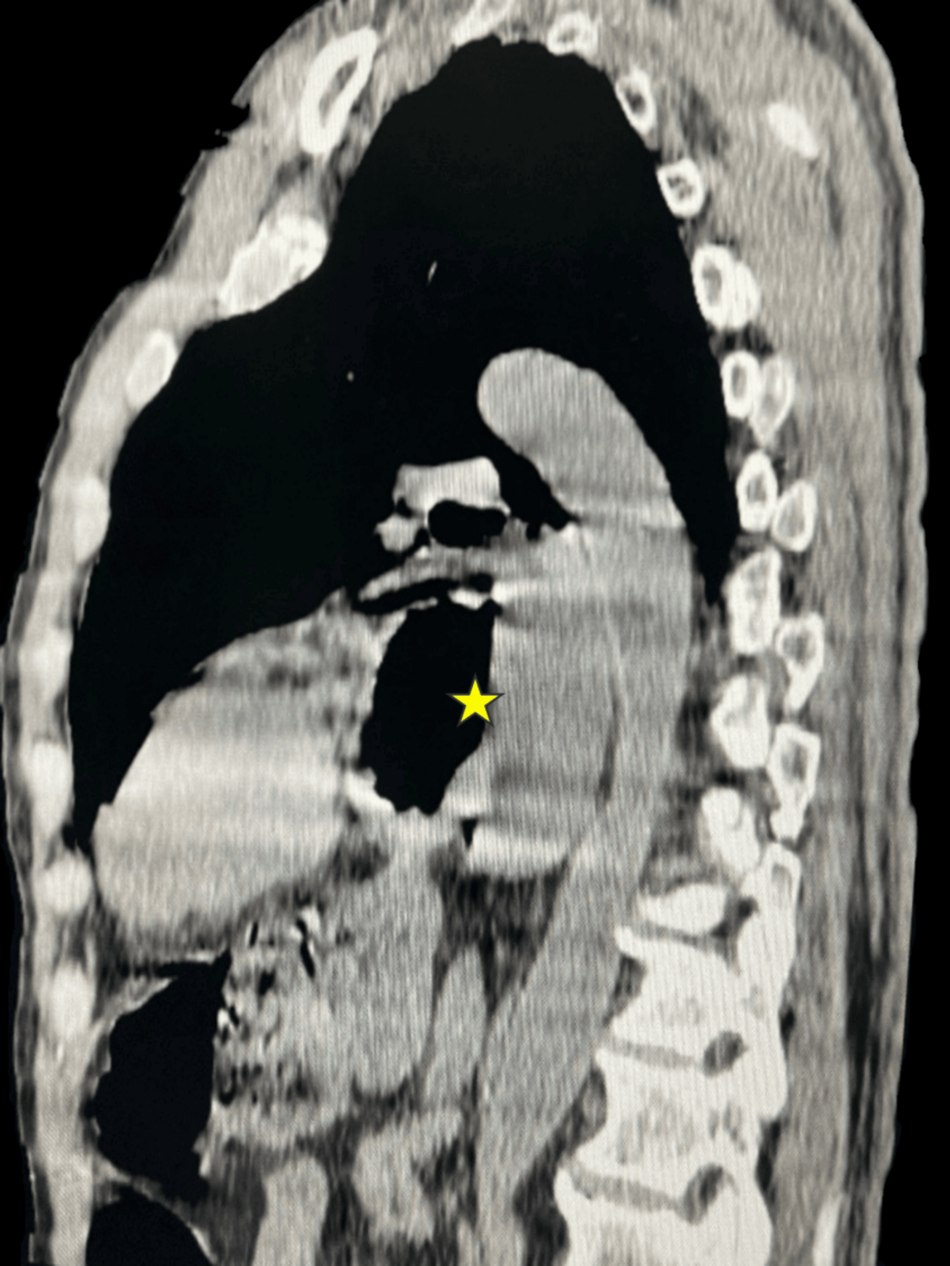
Sagittal reconstruction ‎of thoracic computed tomography ‎ Herniation of the stomach through the esophageal hiatus with abnormal gastric orientation (asterisk).
